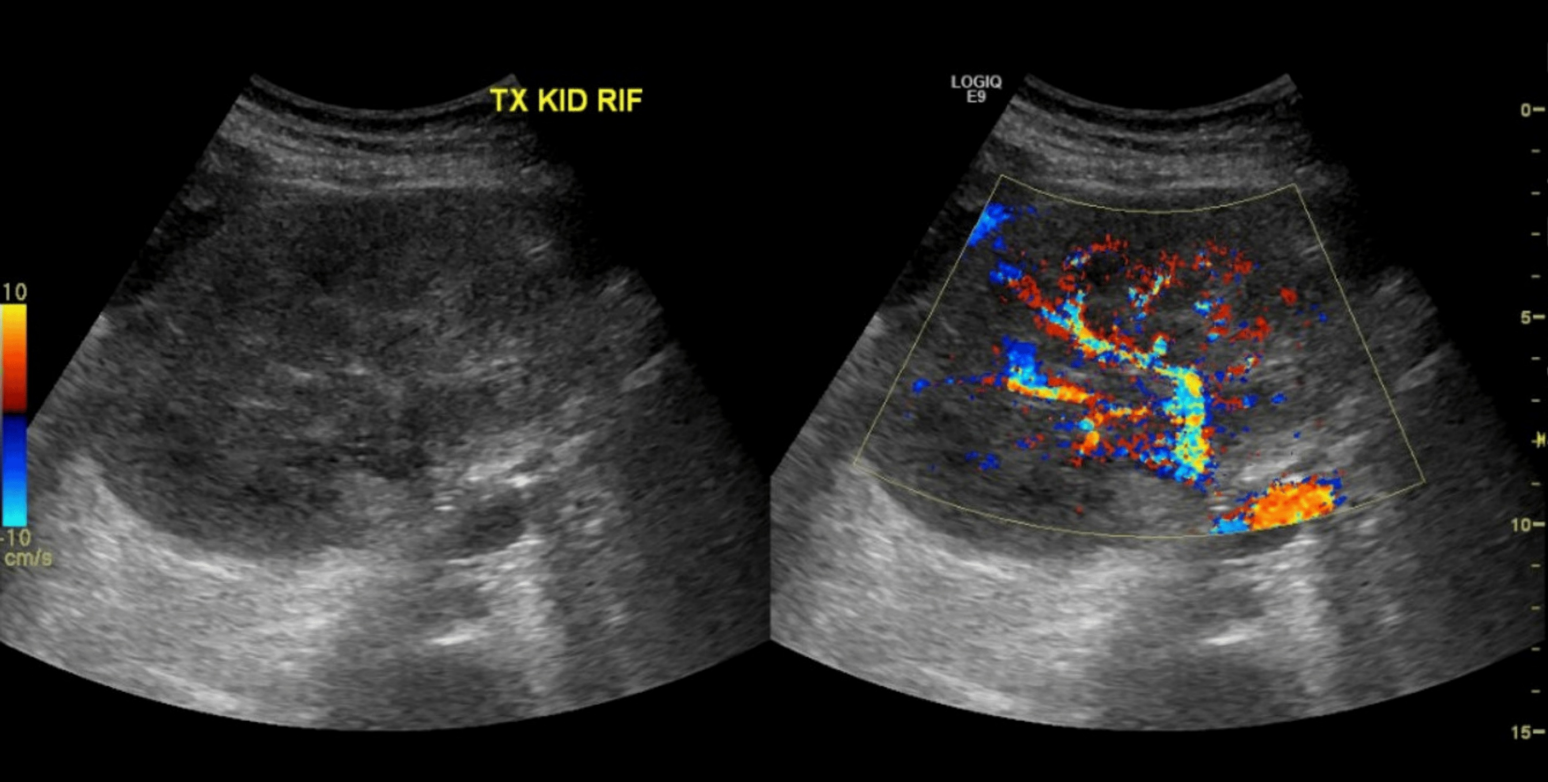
Case 1: Abdominal ultrasound The transplanted kidney, located in the right iliac fossa, is enlarged, measuring 13.7 × 6.3 × 7.2 cm, with an approximate volume of 326 cc.
